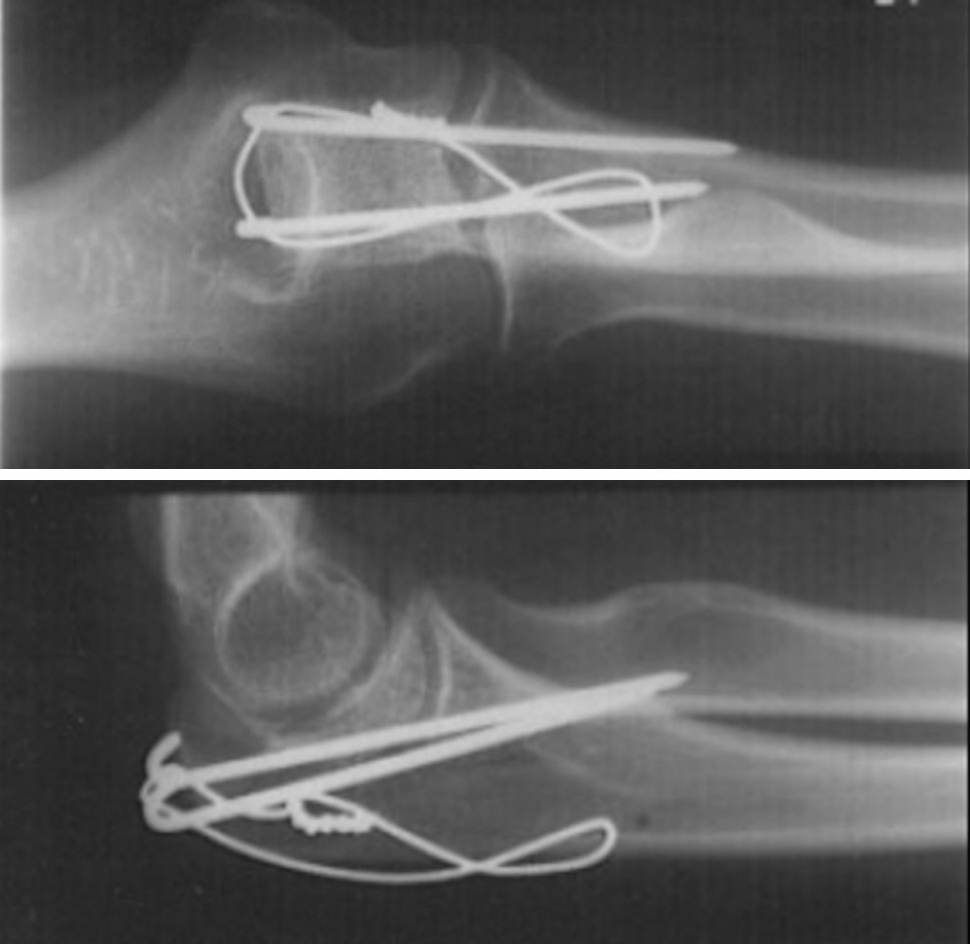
Antero–posterior and lateral radiograph of a simple olecranon fracture. It can be conflicting if twisted ends of the wire knot were sufficiently bent back into direct contact with the bone
